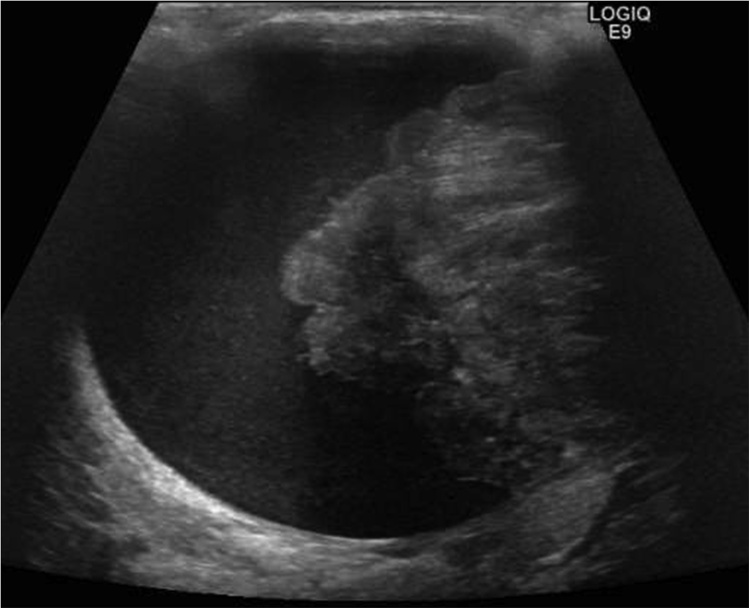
Ultrasonography shows a cystic mass 9 cm in diameter, with a recognizable papillary solid component inside.
